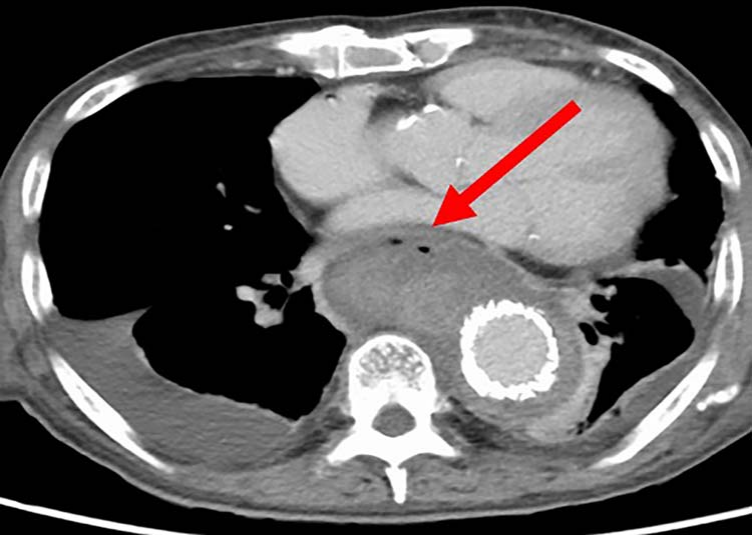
CT seven days after TEVAR. Arrow shows gas images inside the aortic aneurysm.
